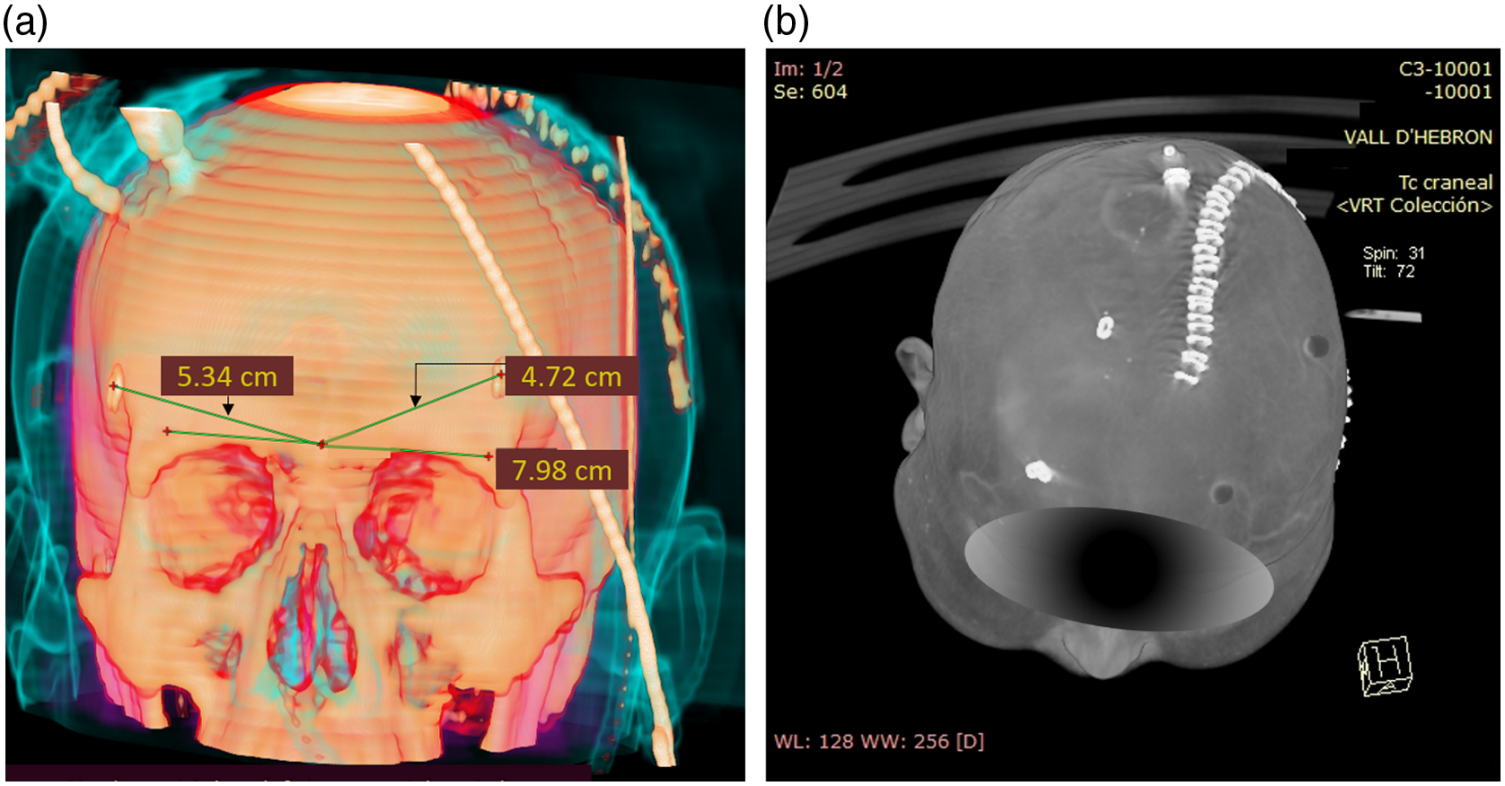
Examples of three-dimensional reconstruction of CT scans with fiducial references of the probes’ placements for two different subjects and measurements. (a) The distances calculated according to the procedure explained in the text are shown. However, it should be considered that these distances are actually shorter than reality, due to the fact that the software did not provide a way to follow the curvature on the skin, whereas it calculates the straight distance between points. (b) The very first trial for recording the positioning of the probes is presented. Two positions were marked by staples, whereas D vitamin capsules were used in the other two. Higher contrast was found for the staples, without producing huge artifacts on tissue and were therefore chosen for the study.
